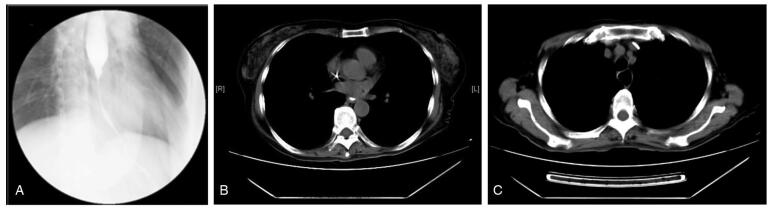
Figure 2. Upper gastrointestinal radiography and computed tomography showed esophageal stenosis. A filling defect in the middle and lower esophagus, stiffening of the wall, and narrowing of the lumen (A). Esophageal stricture was seen in the middle and lower esophagus (B). The cavity of the upper esophagus was dilated. (C).
